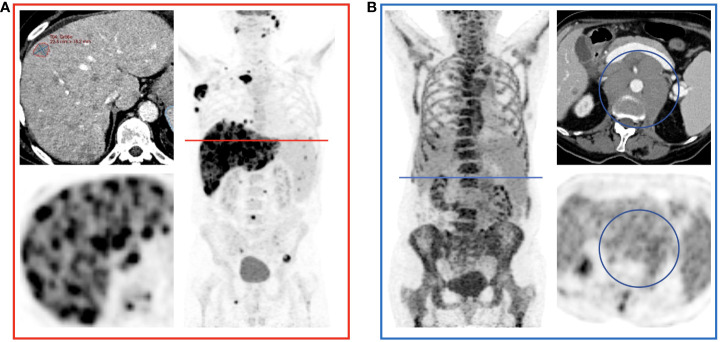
Examples of Over- and Underestimation with Metabolic Tumor Burden as Reference. PET/CT images of the patients color-coded in Figure 2 are shown. (A) shows a patient with disseminated hepatic lymphoma manifestation in whom the tumor burden is underestimated by RECIL and Lugano compared to MTV due to the limited number of target lesions. (B) shows a patient after 4 cycles of bridging therapy in whom only small portions of the retroperitoneal lymphoma manifestation are hypermetabolic. Therefore, compared to MTV, a relatively high tumor burden is detected according to the RECIL and Lugano criteria.
